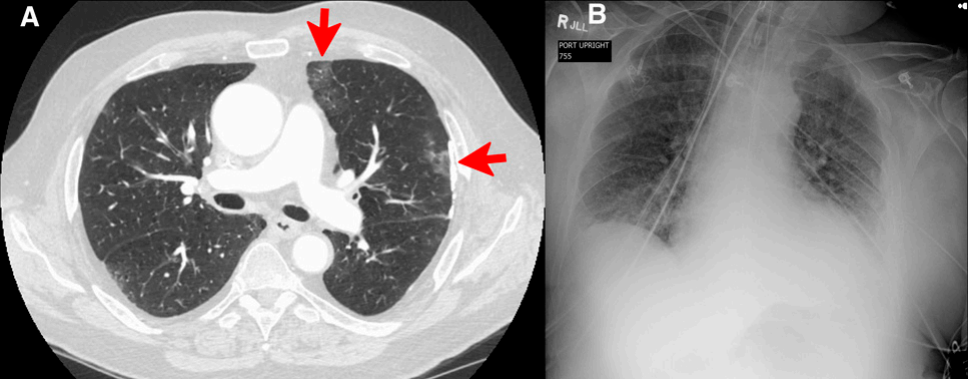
Computed tomography pulmonary angiography at the patient’s initial presentation revealed patchy ground-glass opacities in the upper lobes (red arrows, A). Chest X-ray 2 days before death revealed bibasilar airspace opacities, especially prominent within the left lung base and obscuring the left heart border, with decreased left lower lobe aeration (B). Courtesy of M. B. Burch at the University of Cincinnati.
